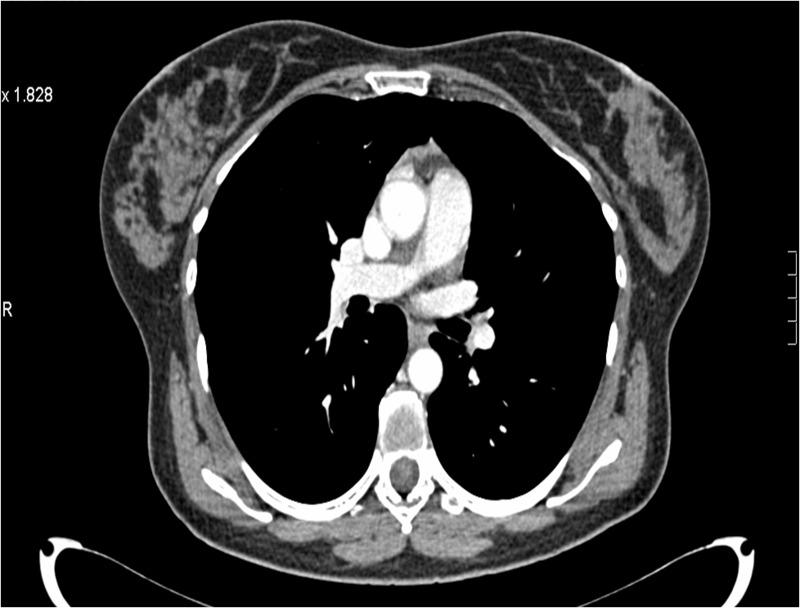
Patient 1: Post-treatment computed tomography (CT) shows resolution of left breast mass
